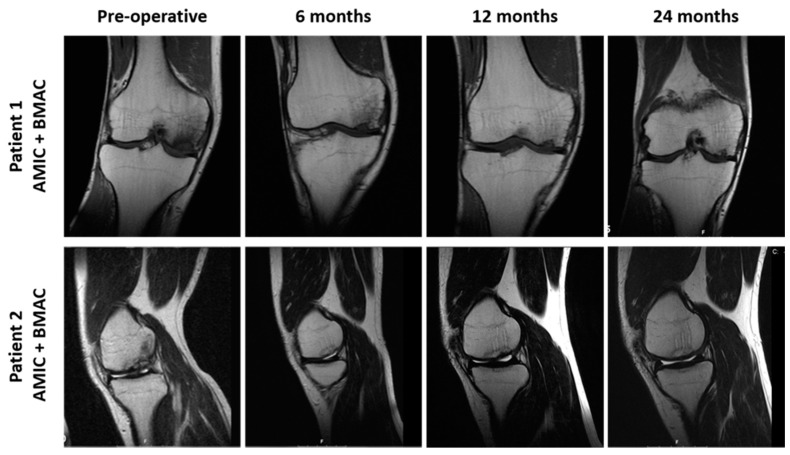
Magnetic resonance imaging (MRI) T1 scans of two patients performed pre-operatively and at 6, 12 and 24 months after AMIC+ treatment.
